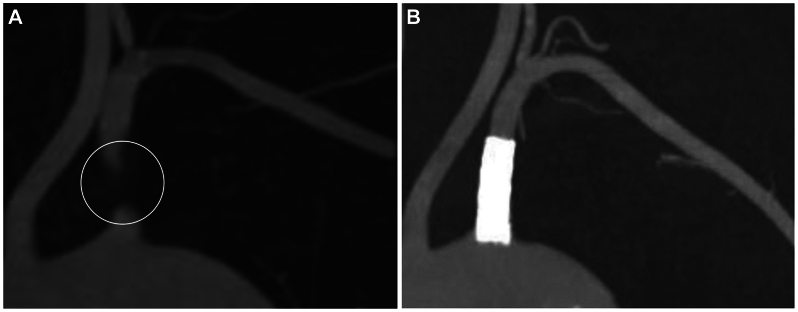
Computed tomography (CT) angiography with the arm dropped. Before (A) and after (B) the endovascular dilation with a stent.
